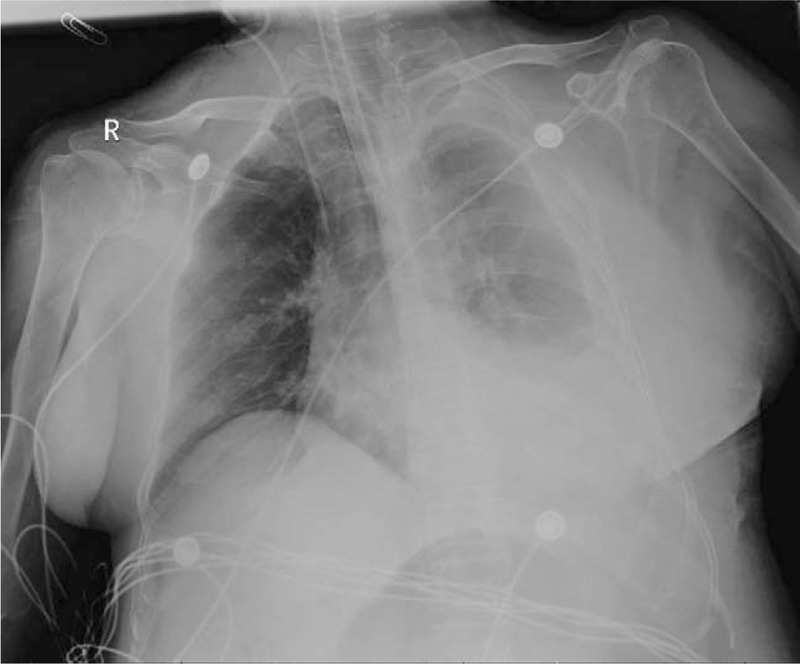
The second chest X-ray displaying a left thoracic hemorrhage.
